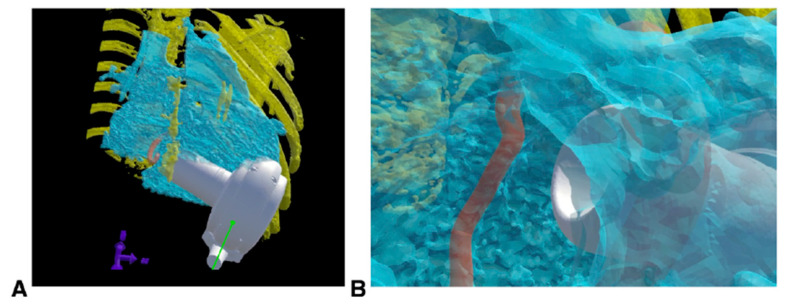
Computed tomography 3D reconstruction of the HeartMate 3™ to assess for device fit within the chest cavity. Image courtesy of Davies et al. 2020, JTCVS Techniques [13]. (A) Placement of the ventricular assist device (white) within the reconstructed heart (blue) and ribcage (yellow). (B) View of the tricuspid valve annulus (red) and ventricular assist device (white).
